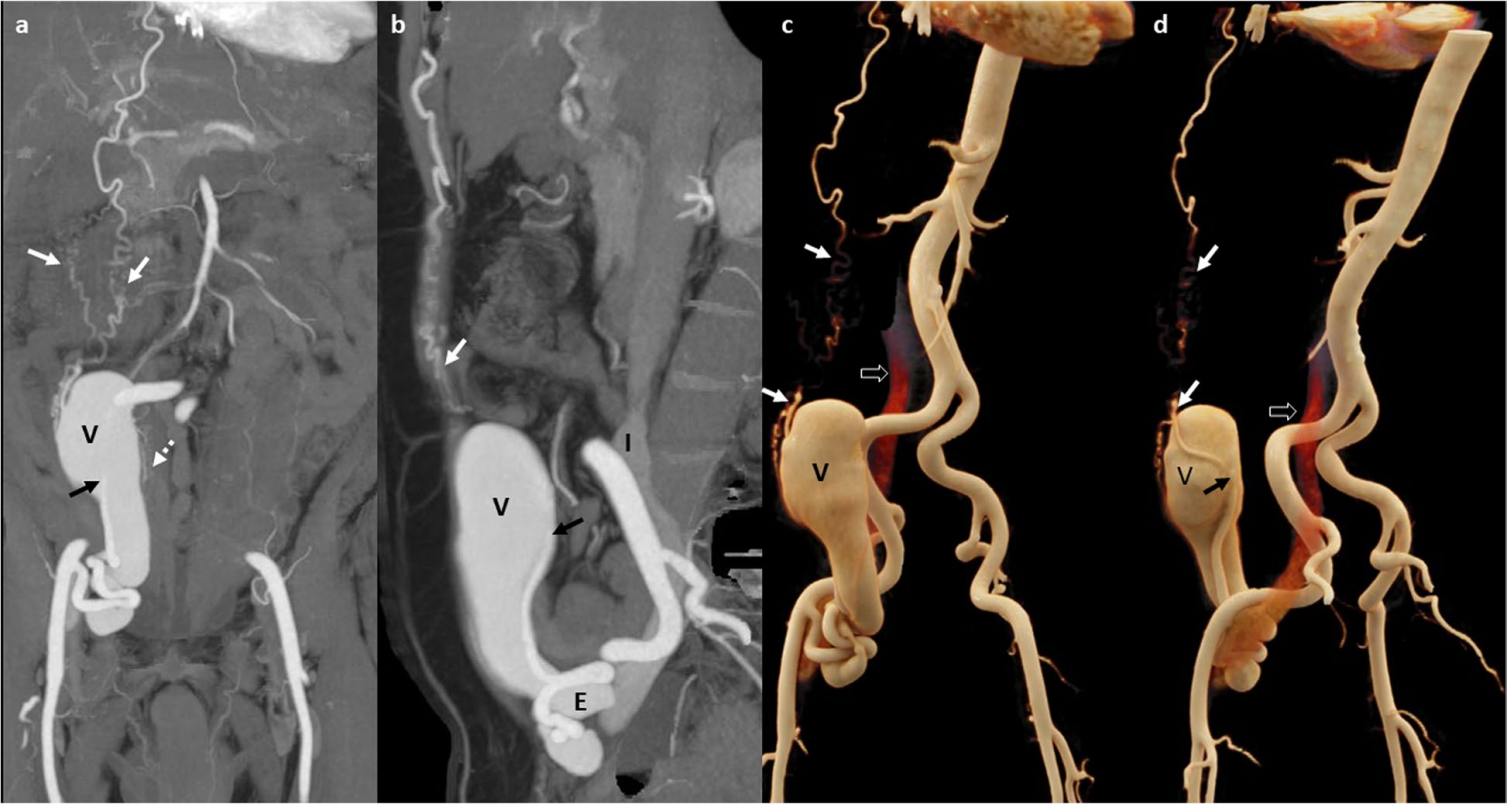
CT angiogram MIP images in coronal (a) and sagittal (b) planes and cinematic VRT images (c anterior and d posterior oblique planes) show dilated and tortuous inferior epigastric artery which is communicating (black arrow) with a large venous sac (V) in right lower abdominal wall, posterior to lower third of right rectus abdominis muscle. Multiple other small arterial feeders from superior epigastric artery (white arrow) and inferior epigastric artery (dashed arrow) are also seen. Early arterial opacification of venous sac (V), external iliac vein (E) and IVC (I) is seen (block arrows in c & d)
